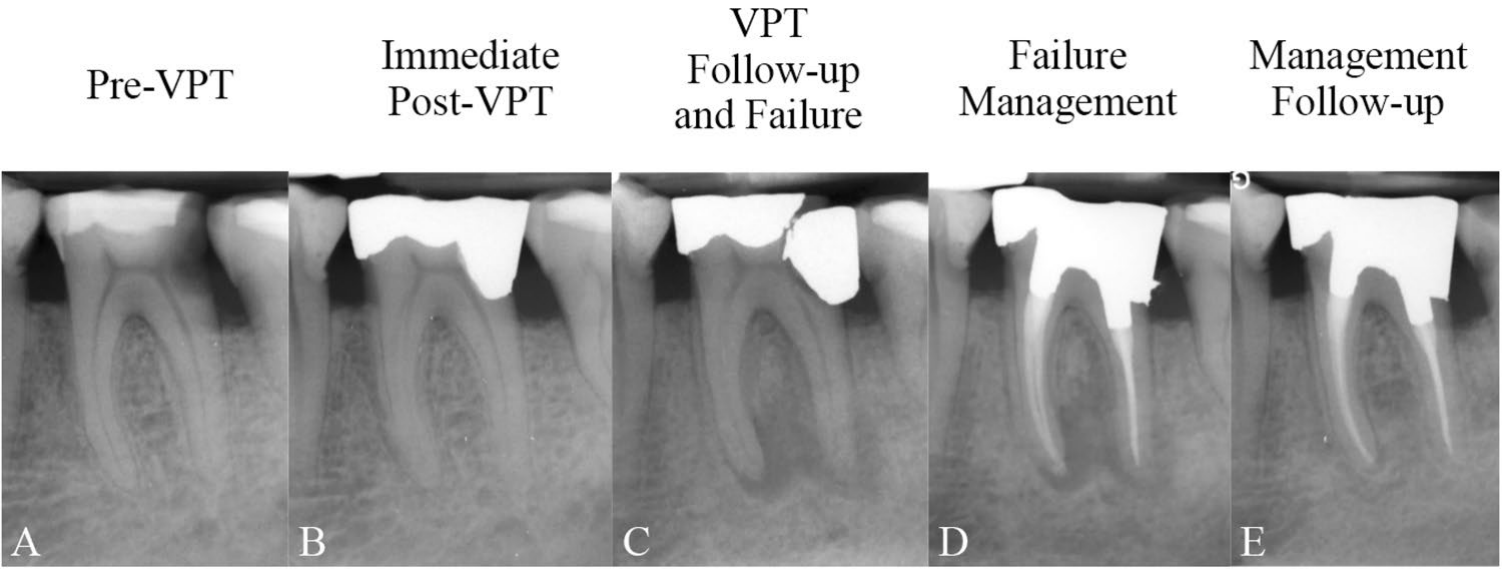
(A) Carious lesion on the lower left first molar of a 60-year-old man diagnosed with irreversible pulpitis; (B) Direct pulp capping and amalgam filling performed; (C) Fracture of the restoration and periapical periodontitis evident at 36-month follow-up, confirming failure; (D) Root canal treatment and amalgam build-up of the tooth completed in a single session; (E) Complete healing of the periapical lesion observed at 27-month follow-up, confirming success.
